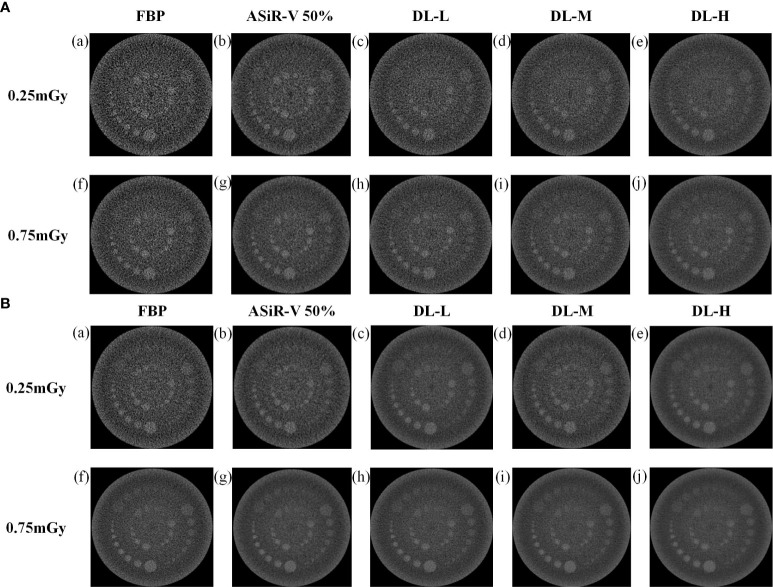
Low-contrast detectability images in axial mode reconstructed with FBP (a, f), ASiR-50% (b, g), and DLIR(L/M/H) (c, h; d, i; e, j) at 0.25mGy and 0.75mGy with a slice thickness of 1.25mm (A) and 5mm (B), respectively. CT, computed tomography; DLIR(L/M/H), deep-learning image reconstruction, level low, medium, and high; mGy, milligray; ASiR-50%, adaptive statistical iterative reconstruction-veo 50%.
